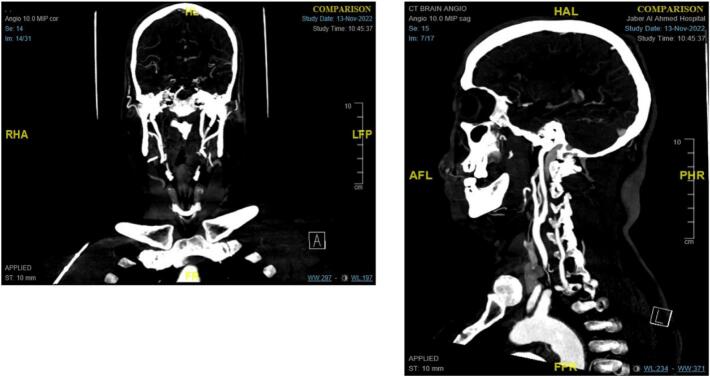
CT angiogram of brain and carotid arteries.
